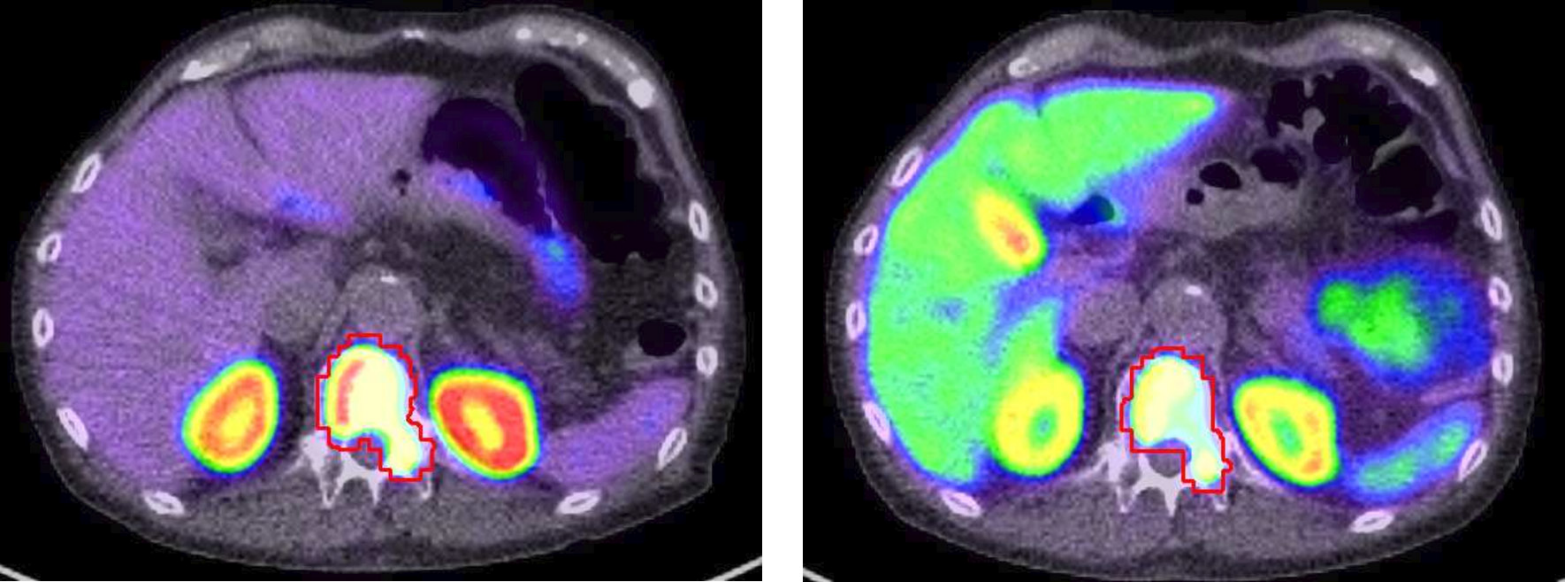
Exemplar PET/CT fusion axial slices taken from the test scan (left) and the retest scan (right) of a single patient using the MIM Encore software. Images show the same osseous lesion in the thoracic spine delineated clearly in red. Patient test scan was acquired using the [68Ga]Ga- PSMA-11 tracer, and retest scan was taken using the [18F]F-PSMA-1007 tracer. PET, positron emission tomography; PSMA, prostate-specific membrane antigen.
